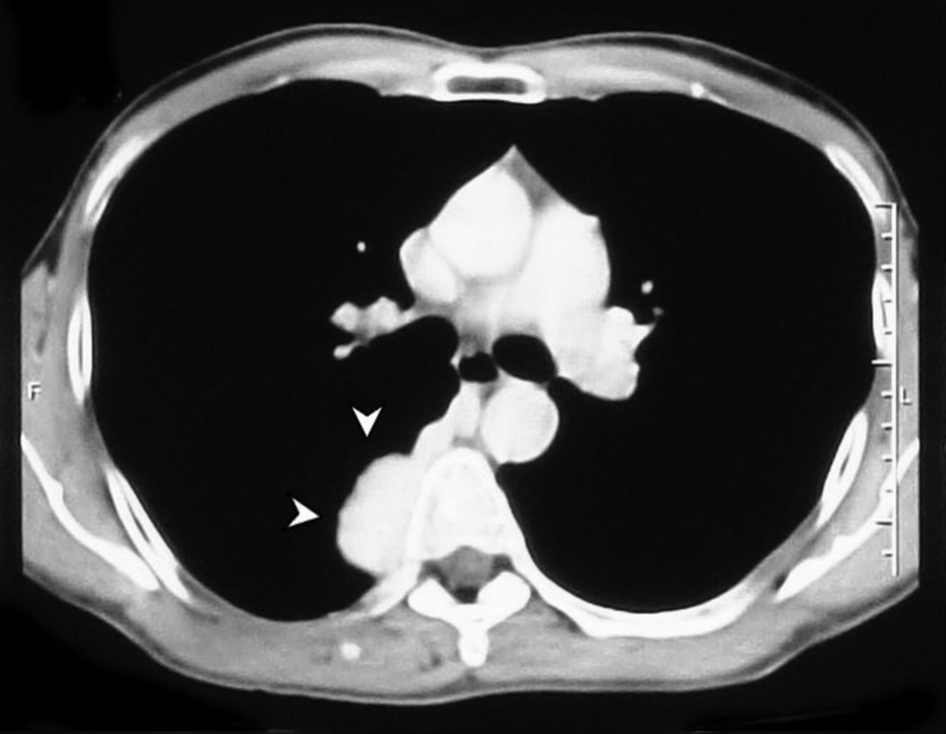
Axial CT scan at the level of the carina shows a lobulated enhancing soft tissue lesion in the right posterior mediastinum
